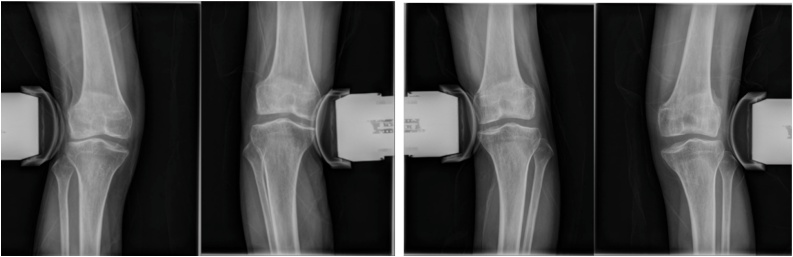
Physician-applied varus/valgus stress radiographs reveals lateral and medial compartment joint opening on the both knee and it indicated instability of both knees. A: Right knee. B: left knee.
